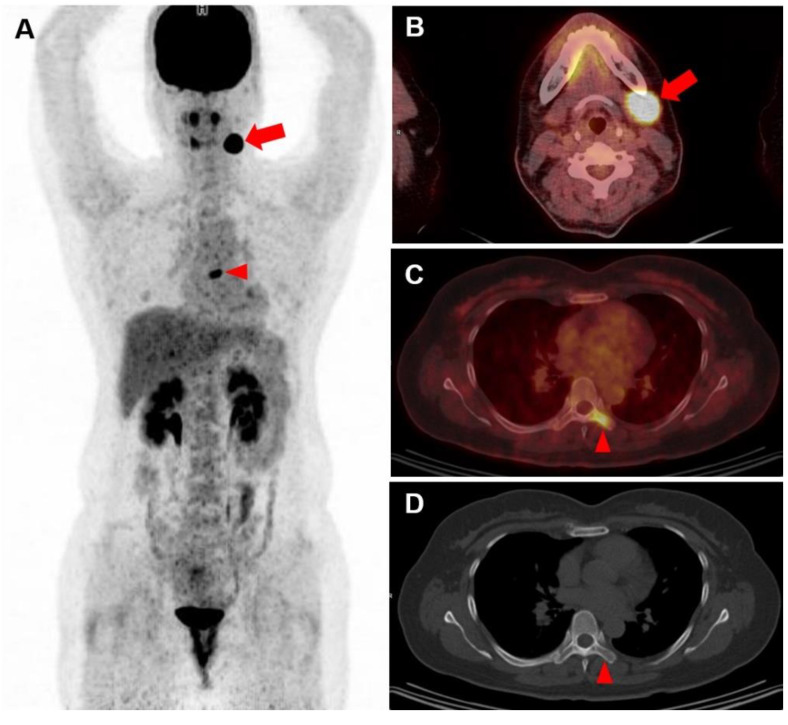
Baseline FDG PET/CT scan of a 48-year-old female patient with DLBCL confirmed by biopsy of left submandibular lymph node. Maximum intensity projection PET (A) and transaxial fusion PET/CT (B) images showed intense localized FDG uptake in left submandibular area (arrows; SUVmax 23.5). In addition, focal FDG uptake was noted in left transverse process and lamina of T6 vertebra (C; arrowhead; SUVmax 6.3), and considered as PET-positive on visual analysis. No abnormal lesion was detected on the corresponding bone setting CT image (D; arrowhead). However, she had BMBx-negative result.
